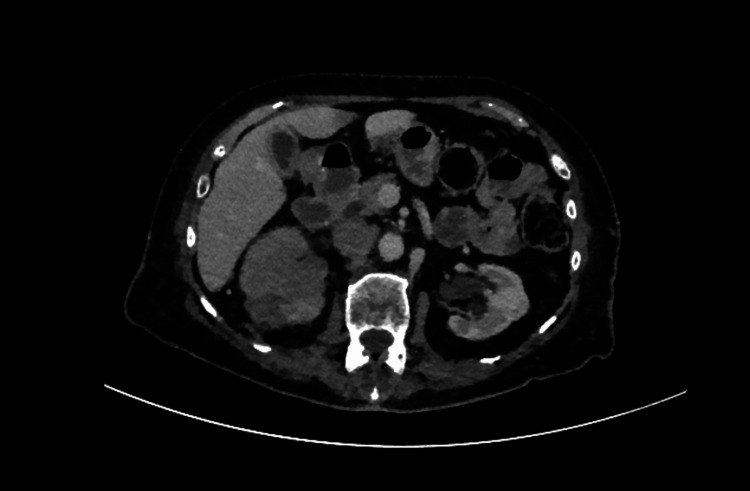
CT demonstrating indeterminate renal findings
